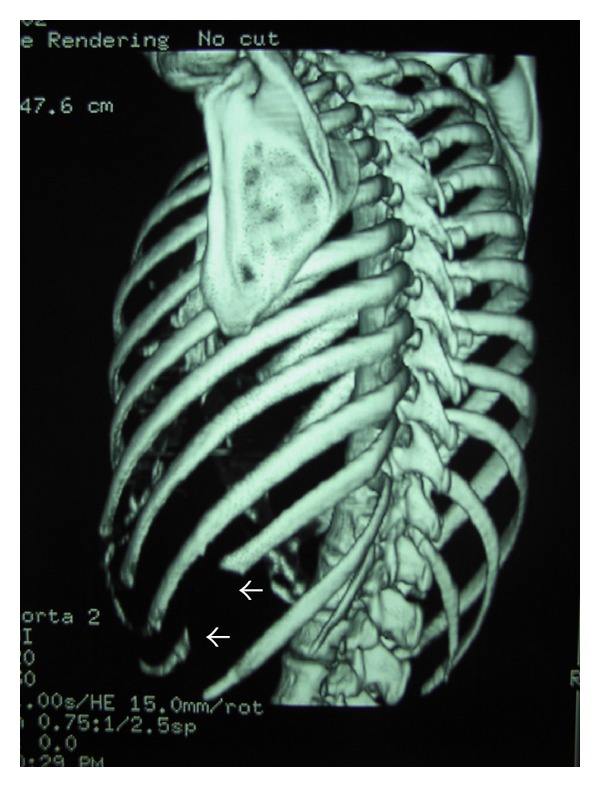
CT (3 years postoperatively), 3D reconstruction of the thoracic skeleton, showing the surgical defect at the middle part of the left 10th rib (arrow).
